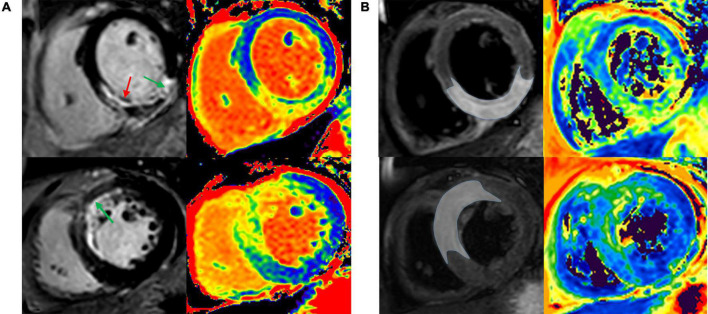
Infarct region and AAR were validated by comparing T1 and T2 mapping against LGE and T2w-STIR. Representative images of patient with acute anterior and anteroseptal STEMI (A), and patient with acute inferior and inferoseptal STEMI (B). AAR, area at risk; LGE, late gadolinium enhancement; T2w-STIR, T2-weighted short tau inversion recovery; STEMI, ST-segment elevation myocardial infarction.
